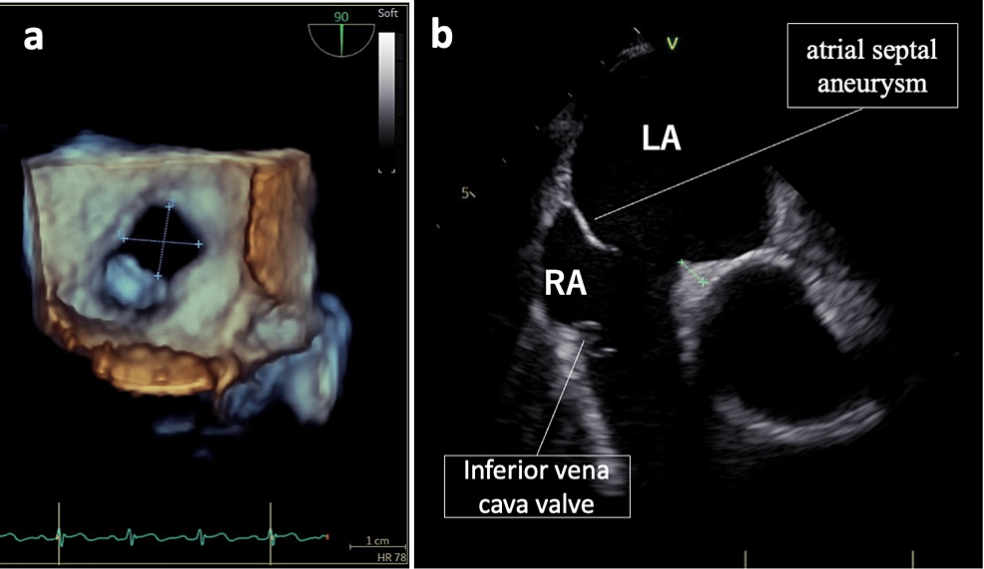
Atrial septal defect detected by transesophageal echocardiography. (a) Transesophageal echocardiography performed in the supine position reveals an atrial septal defect associated with an atrial septal aneurysm. The diameter of the ASD measured is 13 mm. (b). Additionally, an inferior vena cava valve is visualized. LA = left atrium; RA = right atrium
